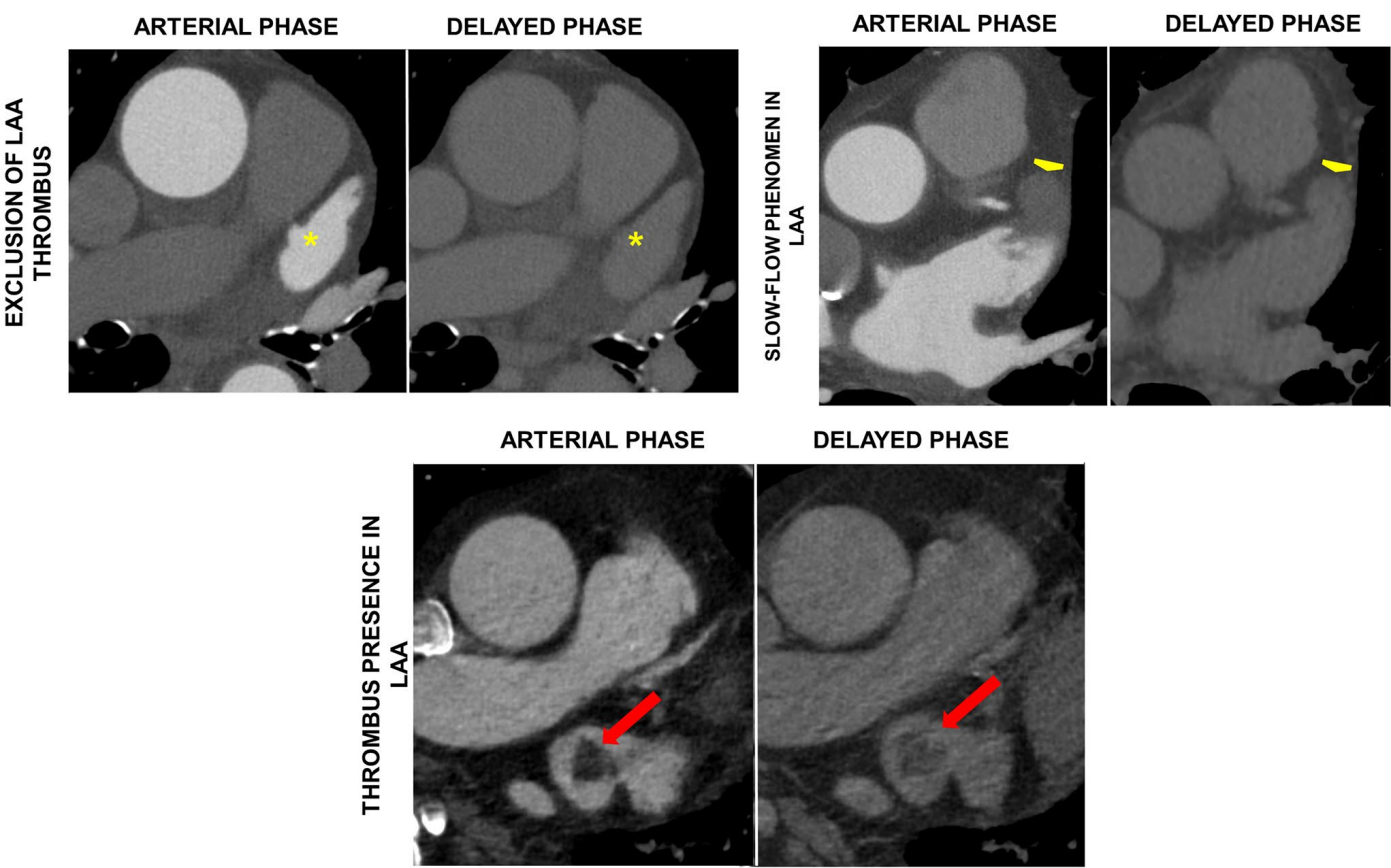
Assessment of LAA using CCT using arterial (left panels) and delayed phase imaging (right panels) in patients with atrial fibrillation planned for pulmonary vein isolation. Upper left panels depicts a representative example of a LAA free of thrombus, evident by the normal contrast enhancement in both phases. Upper right panels demonstrate an example of slow-flow phenomenon in a patient with severely dilated left atrium. Inhomogeneity and lack of contrast enhancement of the distal part of LAA in the arterial phase, which does not persist in the delayed imaging phase, where the LAA is homogenously contrasted. Lower panels depict an LAA thrombus (thrombus in transit) with a filling defect at the proximal part of the LAA in the arterial phase, which persists in the delayed imaging phase. LAA, left atrial appendage, CCT; cardiac computed tomography
